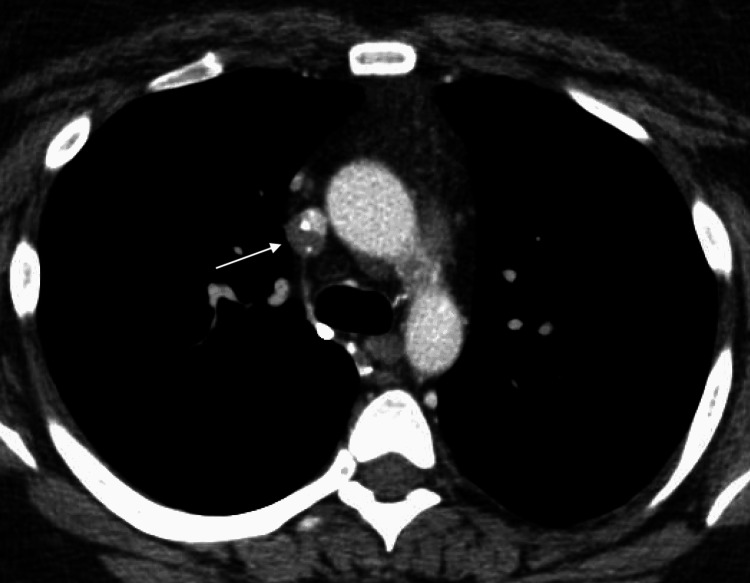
Axial view of a CT chest showing an eccentric, partially calcified, hypodense nodule (1.1 x 1.4 cm, white arrow) abutting and encroaching into the lower SVC CT: Computed Tomography, SVC: Superior Vena Cava
